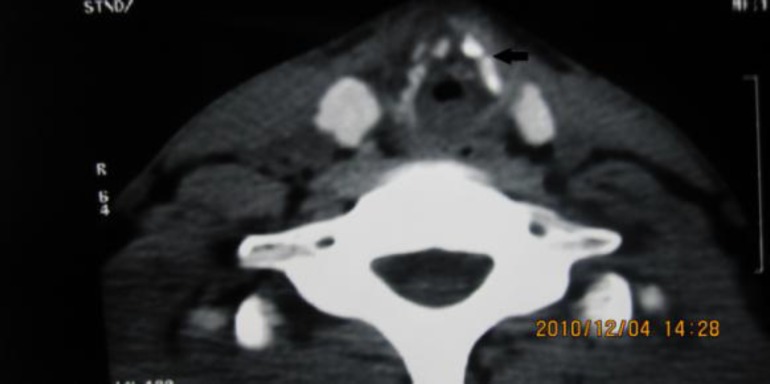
Subglottic airway, pre-op CT scan, showing a distorted airway. Fractured Cricoid cartilage (arrow
